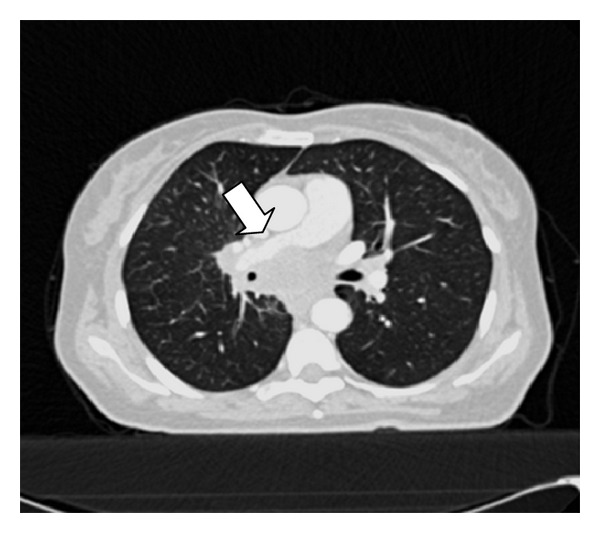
Axial CT image showing well-defined mass lesion causing mild compression of pulmonary artery (arrow).
